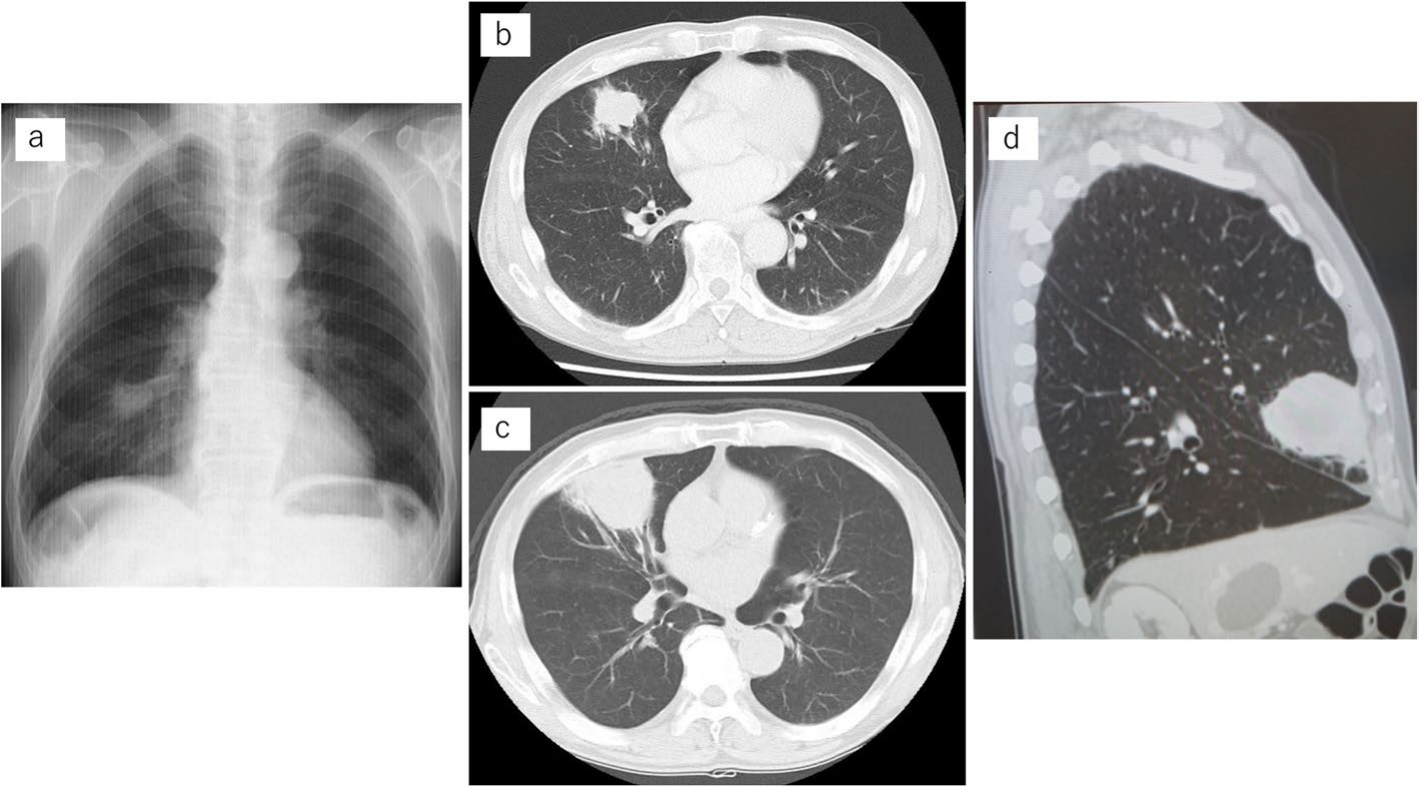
Chest X-ray showed a pulmonary nodule in the right lower lung field (a). Chest computed tomography (CT) revealed a pulmonary nodule 25 mm in diameter in the middle lobe of the right lung (b). Two months later, at the first visit to our University Hospital, CT revealed a rapidly growing tumor 60 mm in diameter (c). CT showed that the interlobar fissure between the right upper and middle lobes was incomplete (d)
